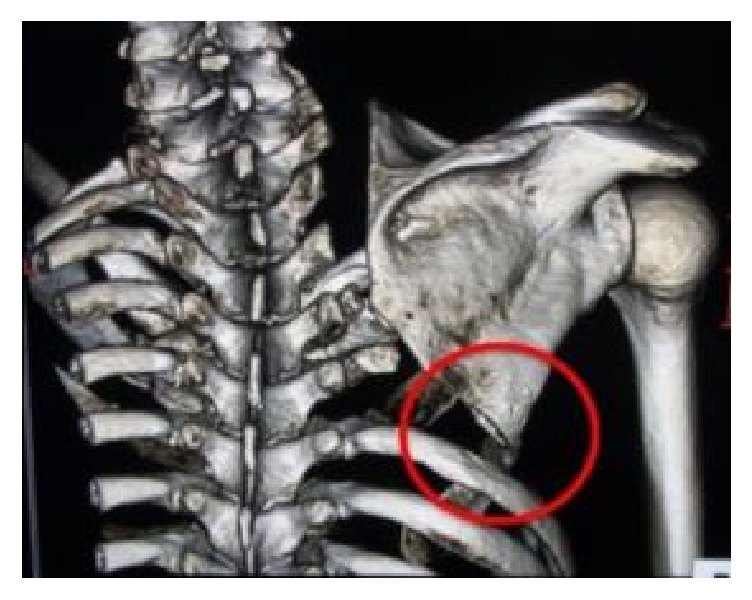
Postoperative CT: locking symptoms improved after resection of the inferior angle of the scapula.
